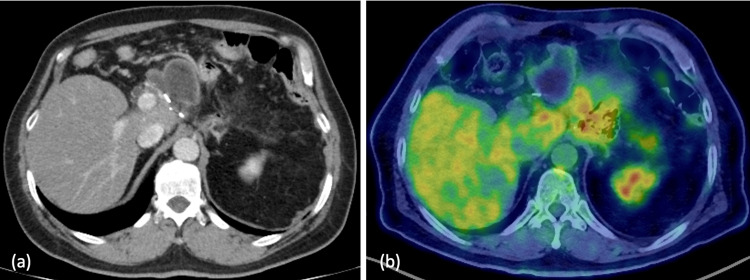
First postoperative CT scan and PET-CT scan (a) Subsequent postoperative follow-up CT revealed the presence of cystic lesions. (b) Subsequent PET-CT imaging indicated the absence of fluorodeoxyglucose (FDG) accumulation and the absence of postoperative recurrence.
